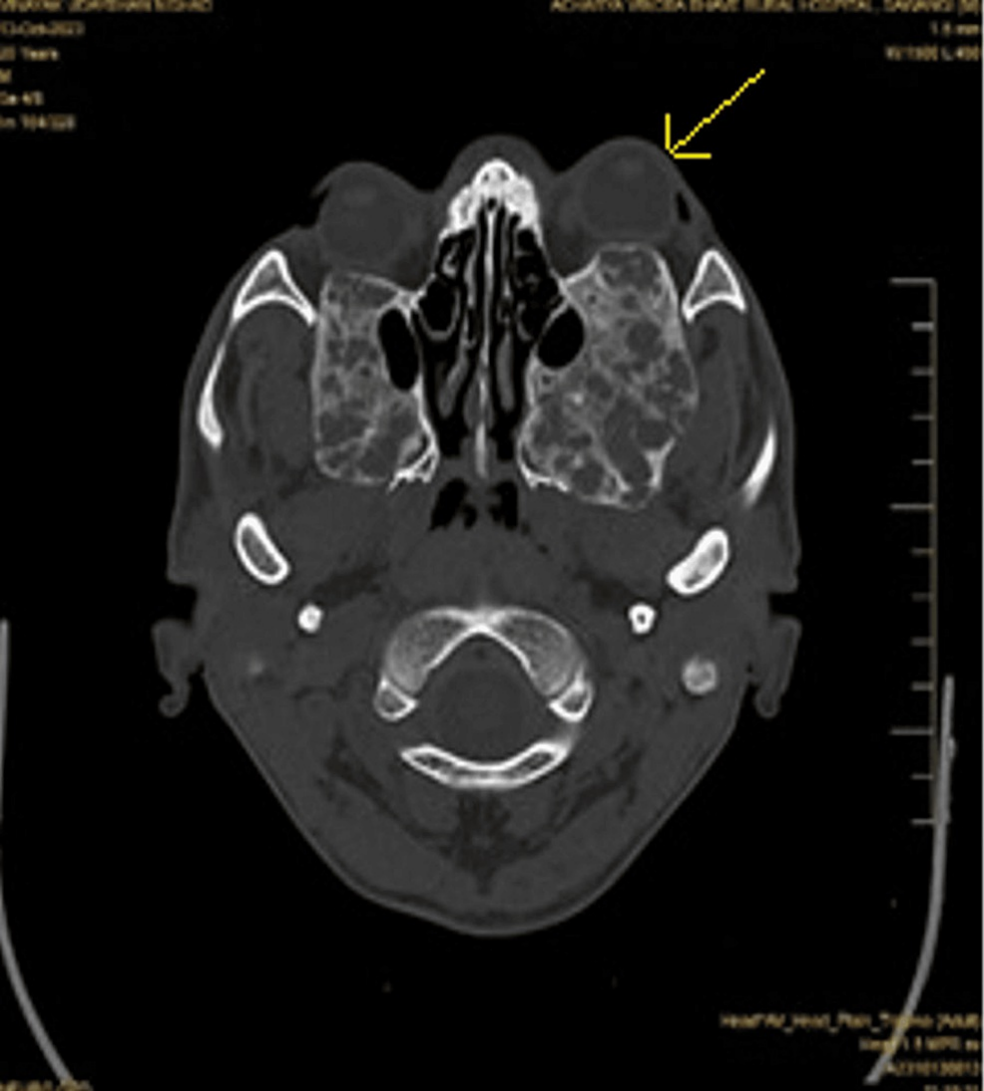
Axial section of CT showing expansion and multilocularity of the lesions
